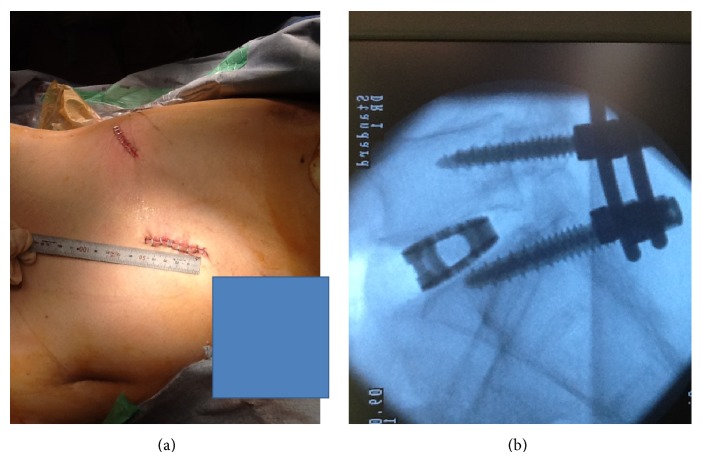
(a) Sutured skin after approaching L5-S1 and harvesting the iliac bone. (b) X-ray image after surgery.
